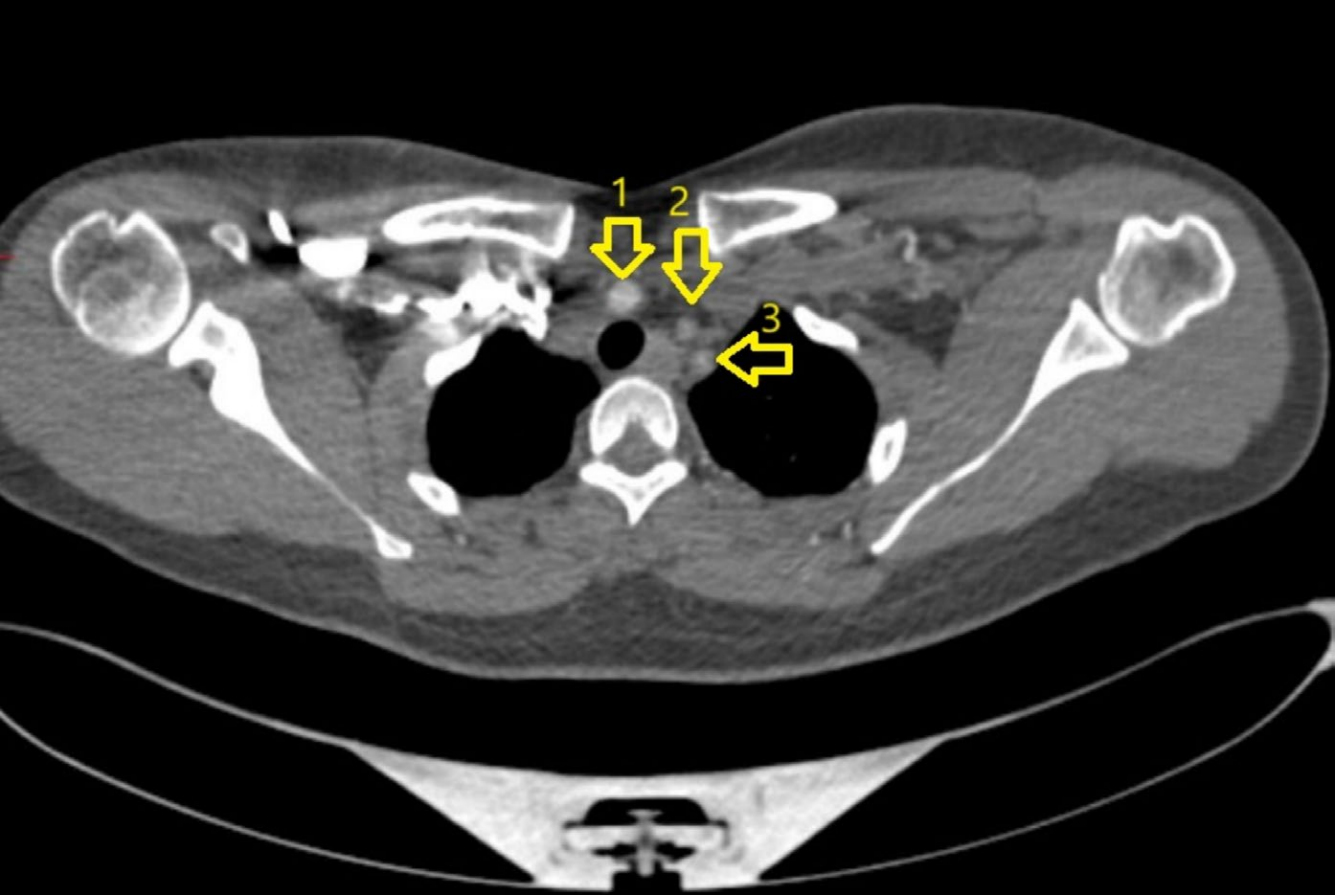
CT‐ axial view demonstrating stenosis of brachiocephalic trunk [1], left common carotid [2] and left sub‐clavian artery [3].
